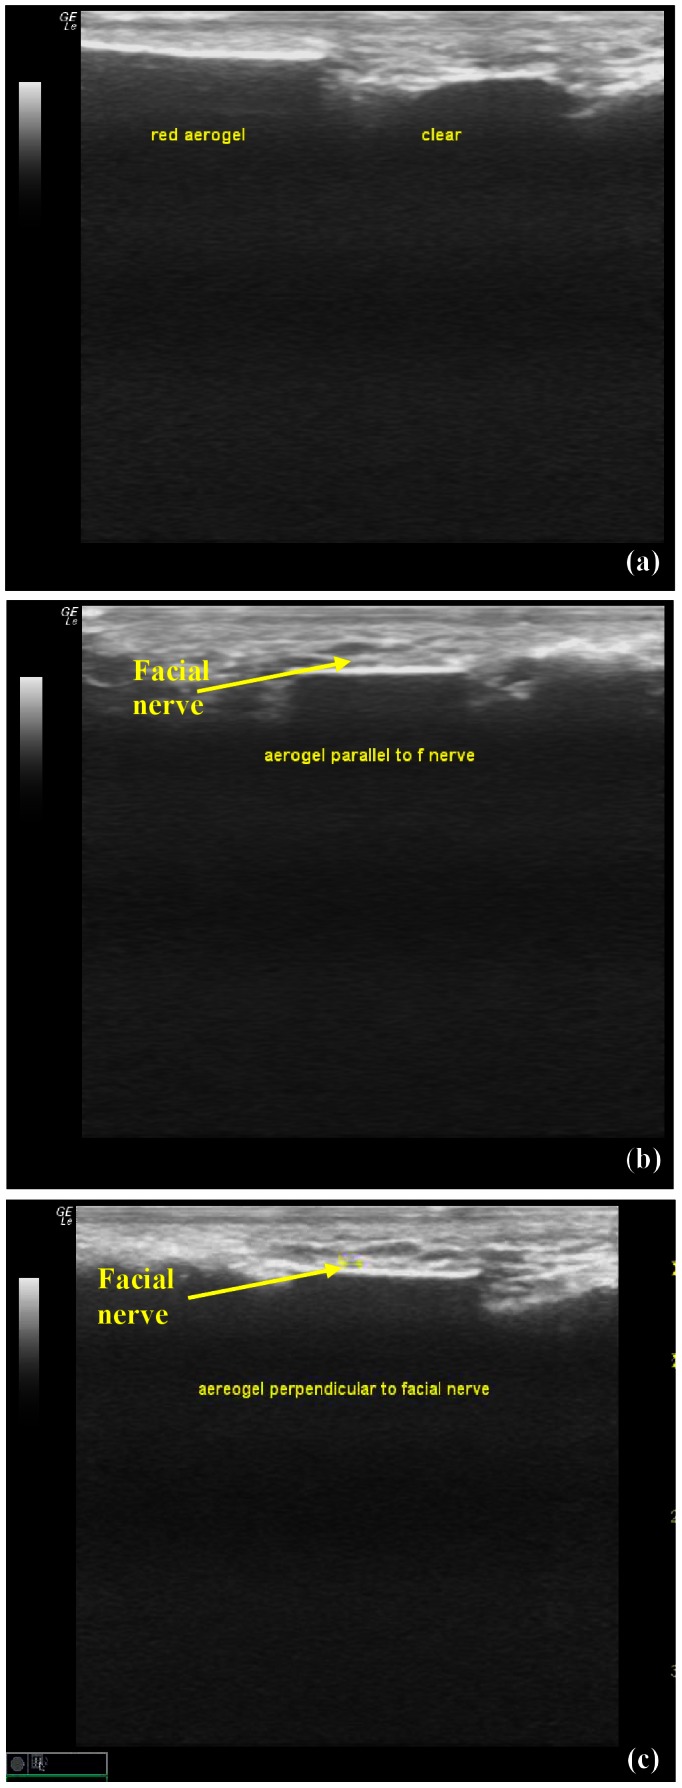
Ultrasonic imaging of subcutaneous X-Si aerogel implants in human cadaver at 13 MHz. (a) Ultrasonic images of pigmented and clear X-Si aerogel implants positioned immediately under the skin, in the parotid region. Some posterior shadowing is observed. The structure of the pigmented (red) and clear aerogels were indistinguishable at the imaged frequency. (b) Parallel and (c) perpendicular orientations of clearX-Si aerogelwith respect to the facial nerve, as indicated by the arrows.
